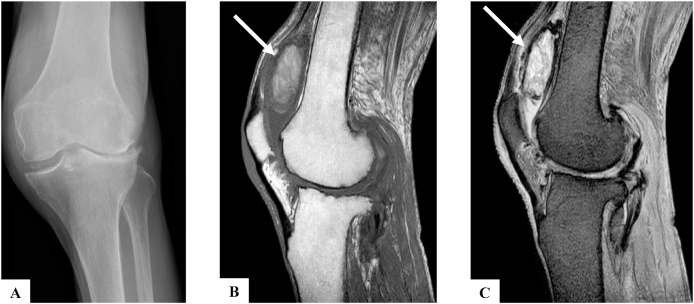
X-ray and MRI findings. A) X-ray showing lateral knee osteoarthritis. B) T1-weighted MRI image showing a hypointense appearance mass in the suprapatellar sac (arrow). C) T2-weighted MRI image showing a hyperintense appearance mass in the suprapatellar sac (arrow).
